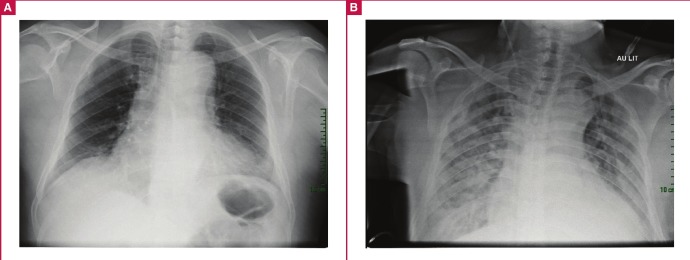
Anterior–posterior chest X-ray. A: At presentation showing enlargement of the mediastinum. B: On day 11 of hospitalisation showing bilateral interstitial heterogeneous opacities.
